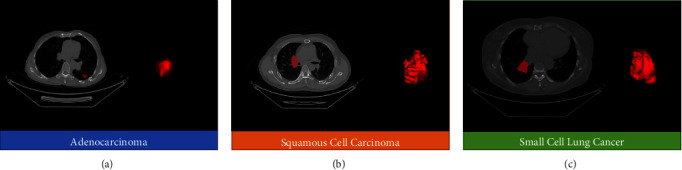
Segmentation of lesions on CT images and 3D ROI for (a) adenocarcinoma, (b) squamous cell carcinoma, and (c) small cell lung cancer.
